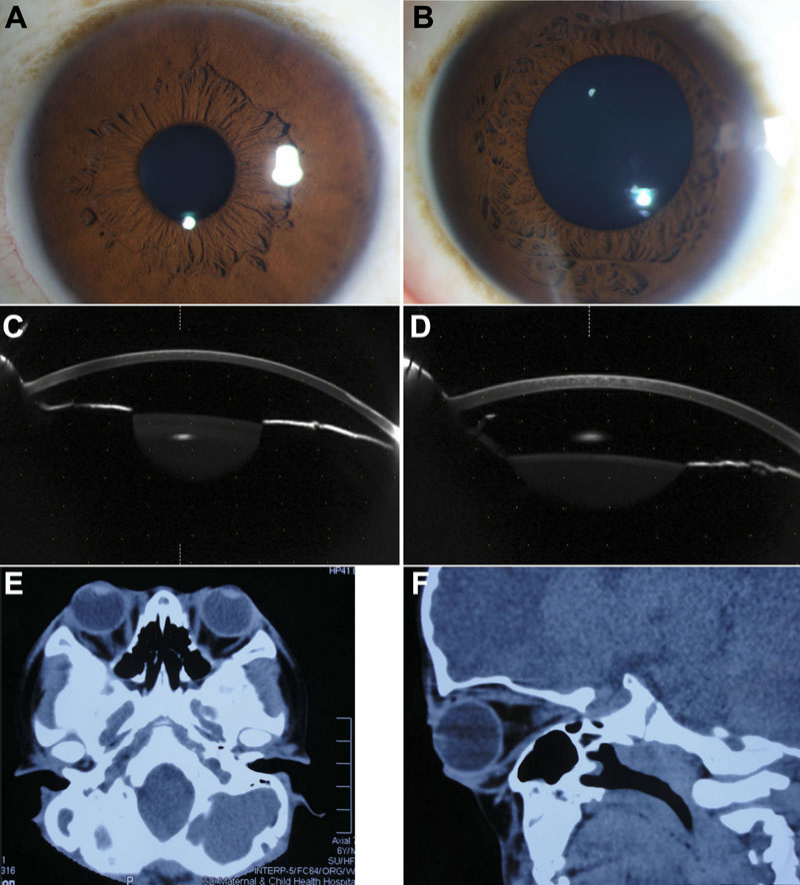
Examination results of II-3 and III-1. A: Anterior segment photograph of II-3. B: Anterior segment photograph of III-1. C: The anterior segment picture of II-3 by Pentacam. D: The anterior segment picture of III-1 by Pentacam. E, F: Shallow orbits and ocular proptosis of III-1 using computed tomography (CT).
